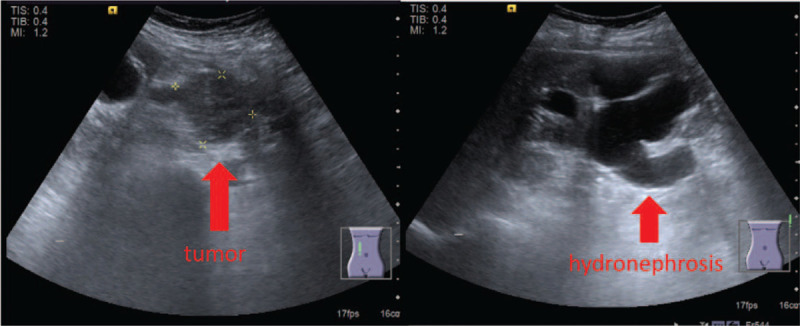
A heterogeneous hypoechoic lesion measuring 4.5 × 3.9 cm was found over the right retroperitoneal cavity by ultrasound, and induced right moderate hydronephrosis.
